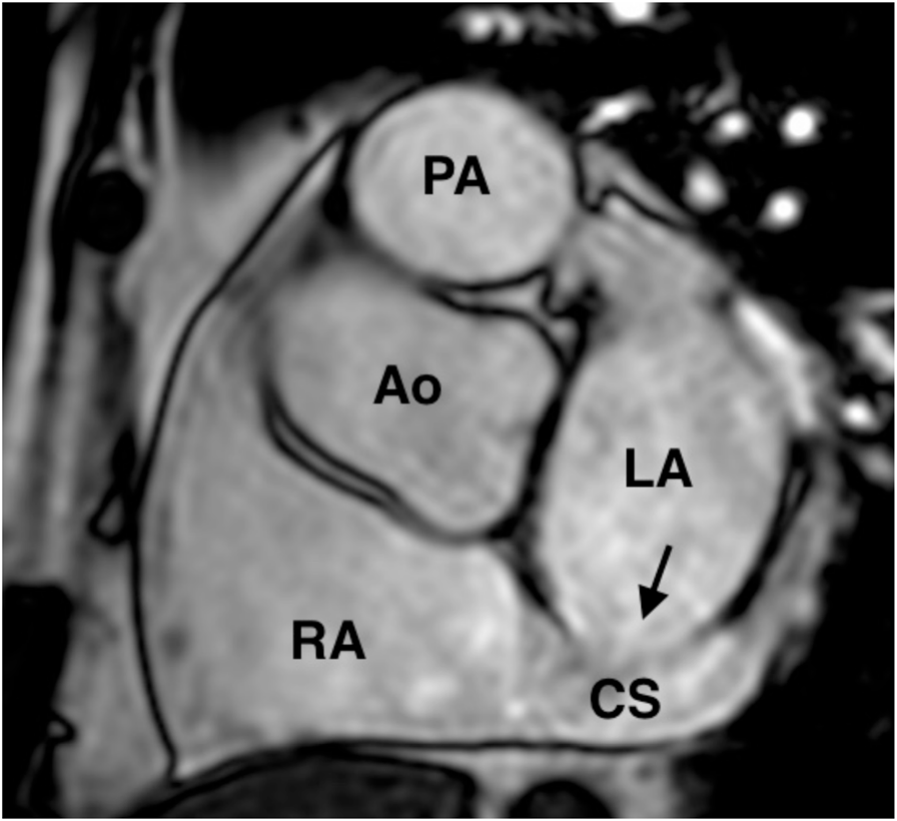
Magnetic resonance imaging in case 4. Magnetic resonance imaging (sagittal view) in case 4 showing the morphology of the patient’s congenital heart disease. We found no persistent left superior vena cava or other congenital cardiovascular anomalies. The arrow indicates the coronary sinus defect. *Ao* aorta, *CS* coronary sinus, *LA* left atrium, *PA* pulmonary artery, *RA* right atrium
